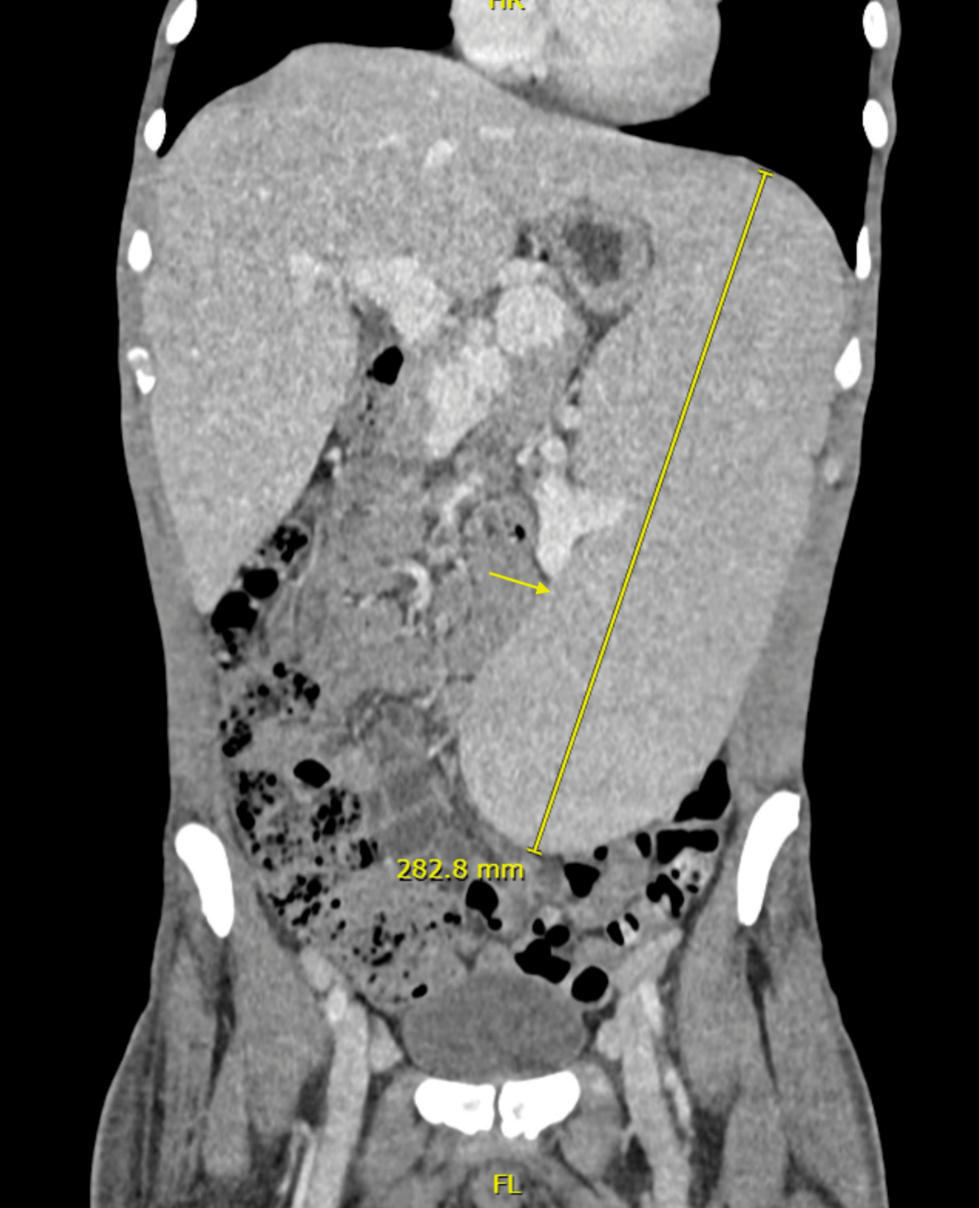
Computed Tomography of the abdomen Massive splenomegaly as indicated by the yellow arrow
